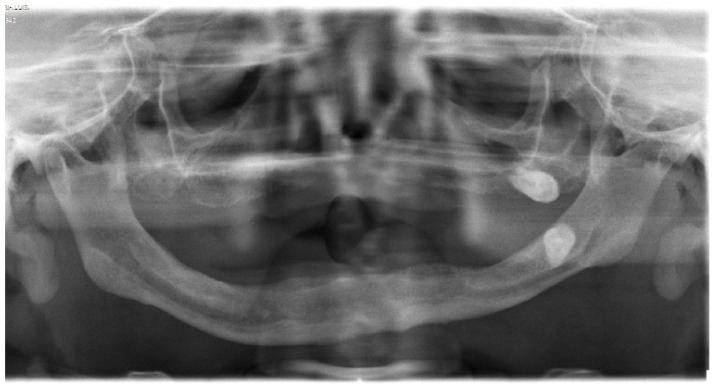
Orthopantomography of patient.
